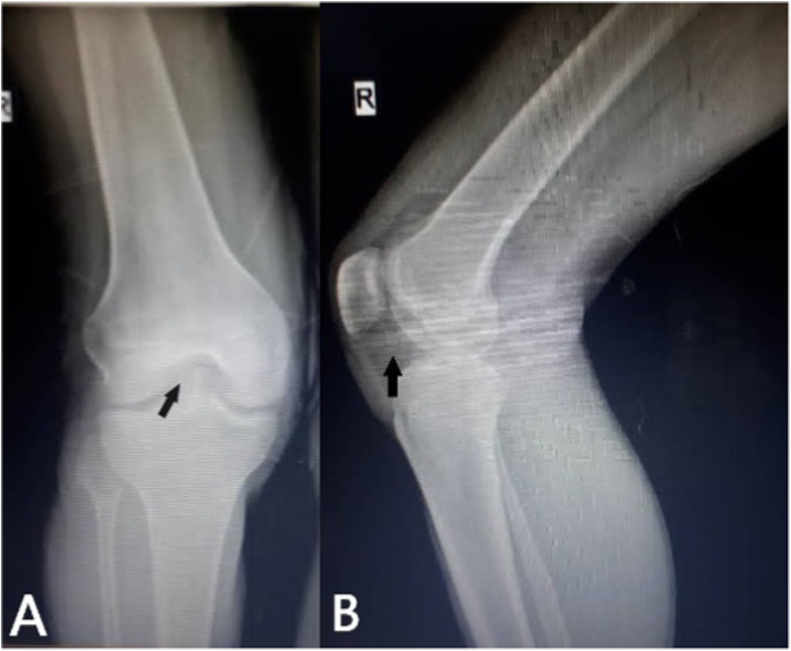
Anteroposterior (A) and lateral (B) X-rays; reveal a shadow into the anterior compartment of the right knee joint (black arrows).
